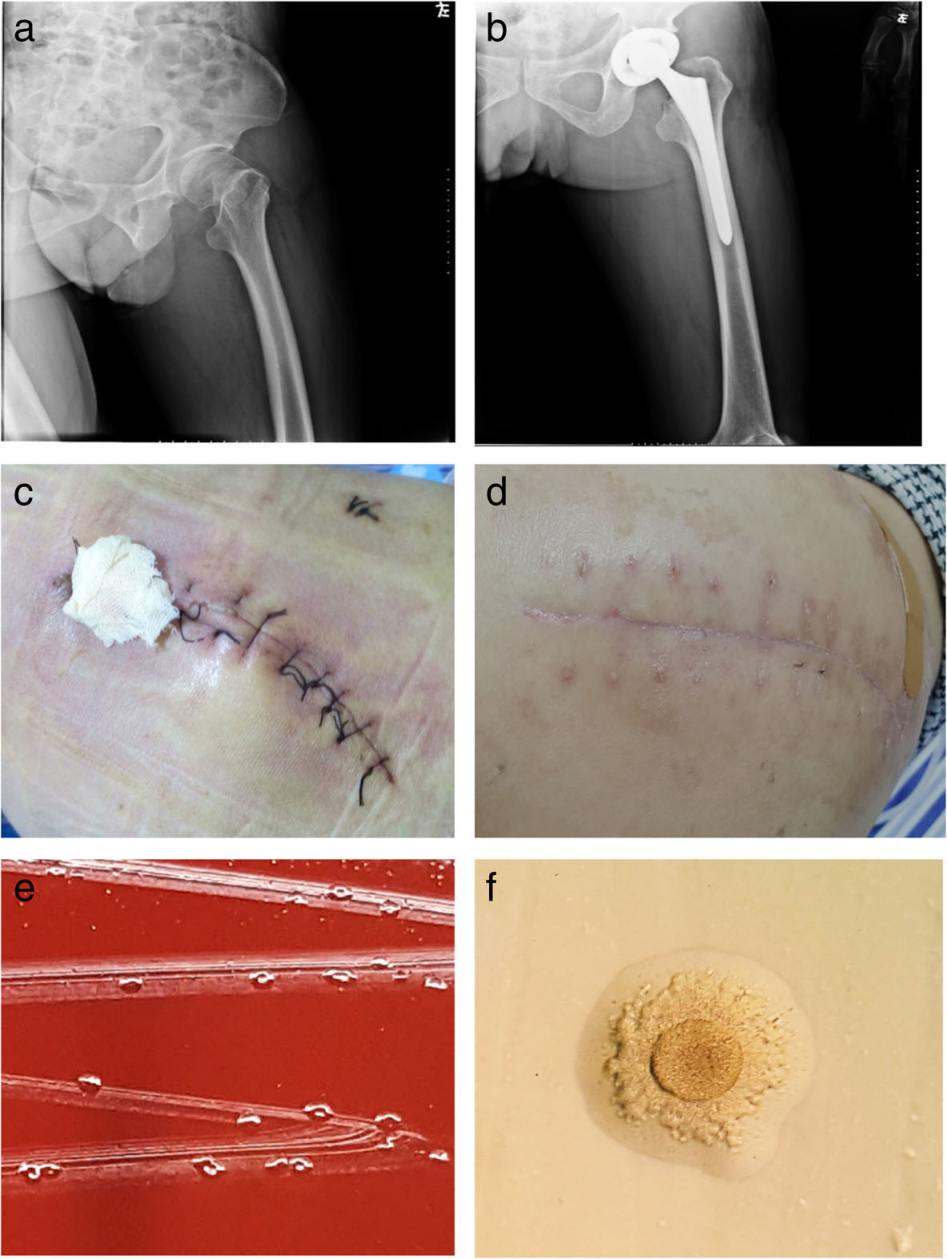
a Fractures of left femoral neck under X-ray at admission. b Prosthetic hip in right position (2 h after hip replacement under X-ray). c Redness and swelling around surgical site, wound fluctuation in palpation (8 days after surgery). d Recovered after anti- *M. hominis* treatment with the combination of moxifloxacin, and doxycycline (18 days after surgery). e After 7 day of incubation of subcutaneous fluid collected during debridement on blood agar at 37 °C in a 5% CO_2_ atmosphere, tiny, nonhemolytic, transparent colonies grew on Columbia blood agar plate. f Fried-egg-type colonies of *Mycoplasma hominis* on solid media 5 days after subculture
